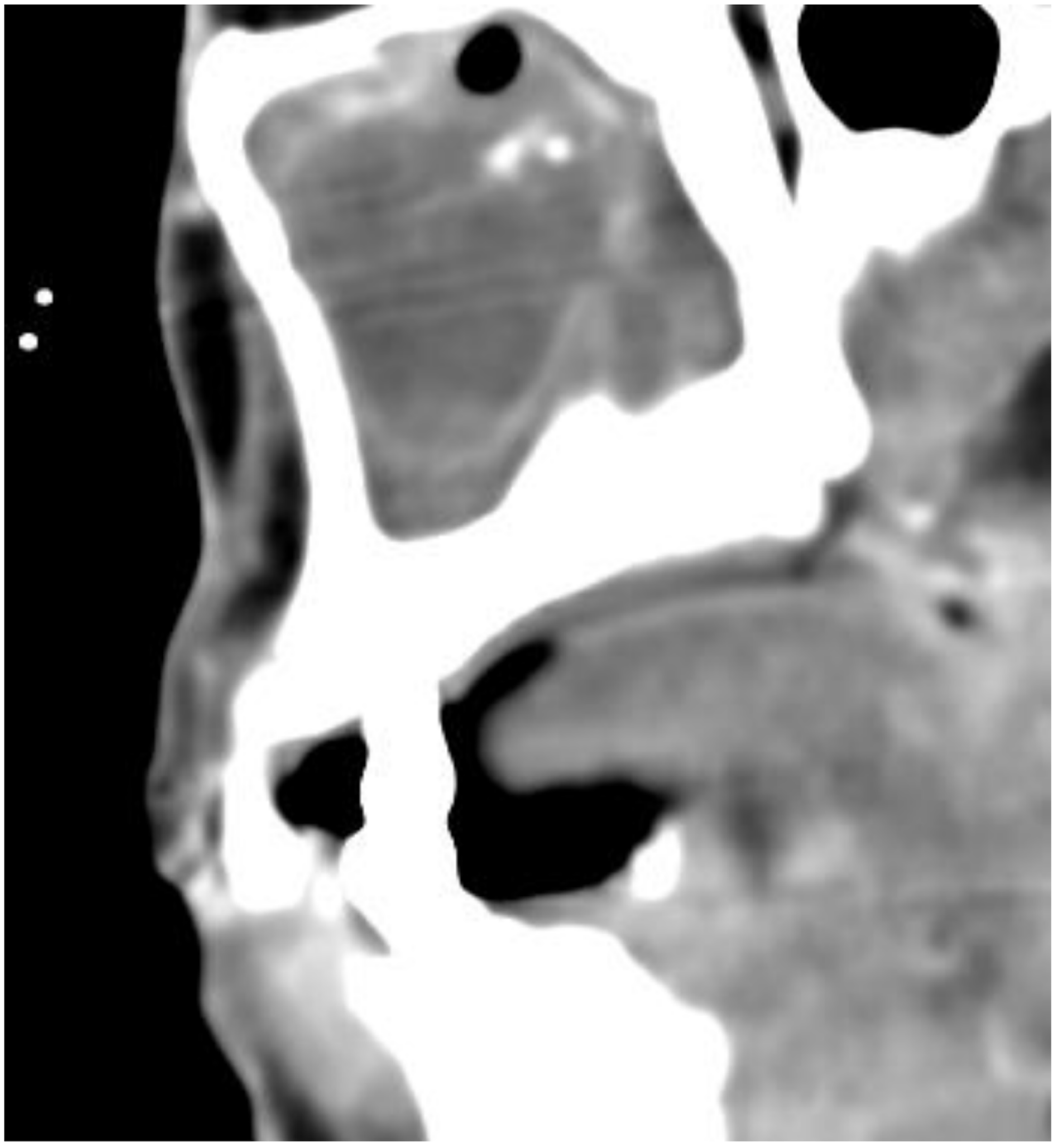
CT-scan in a sagittal section showing a mucosal swelling and obstruction of the maxillary sinus due to an aspergilloma (Contrast medium enhancement).
